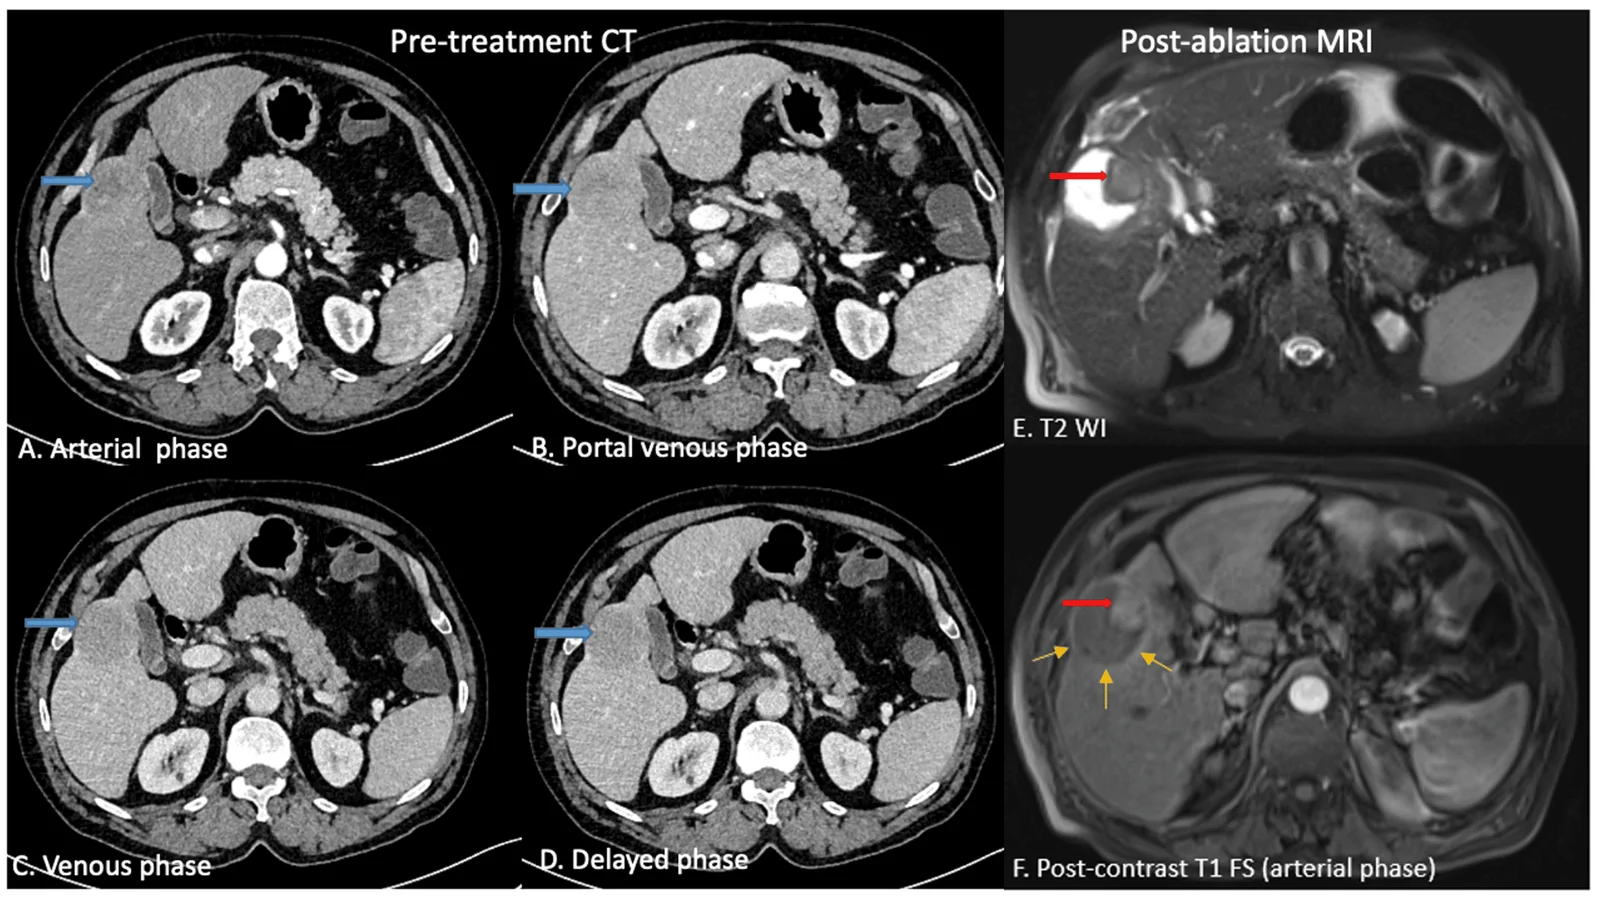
Pretreatment contrast-enhanced CT and post-microwave ablation contrast-enhanced MRI demonstrating an LR-TR Viable observation. Pretreatment axial multiphasic contrast-enhanced CT images obtained during the arterial (A), portal venous (B), venous (C), and delayed (D) phases demonstrate a well-defined hepatic observation measuring >2 cm in segment V (blue arrow). The observation demonstrates nonrim arterial phase hyperenhancement, nonperipheral washout, and an enhancing capsule, consistent with an LR-5 observation. Post-treatment axial MRI images obtained after microwave ablation, including T2-weighted (E) and post-contrast arterial phase T1-weighted fat-saturated (F) images, demonstrate a treated observation with a nodular mass-like T2 hyperintense focus showing nodular mass-like arterial phase hyperenhancement (blue arrow), consistent with an LR-TR Viable category. A smooth, thin peripheral rim of enhancement surrounding the ablation zone (yellow arrow) represents an expected post-ablation imaging finding and should not be mistaken for viable tumor.
